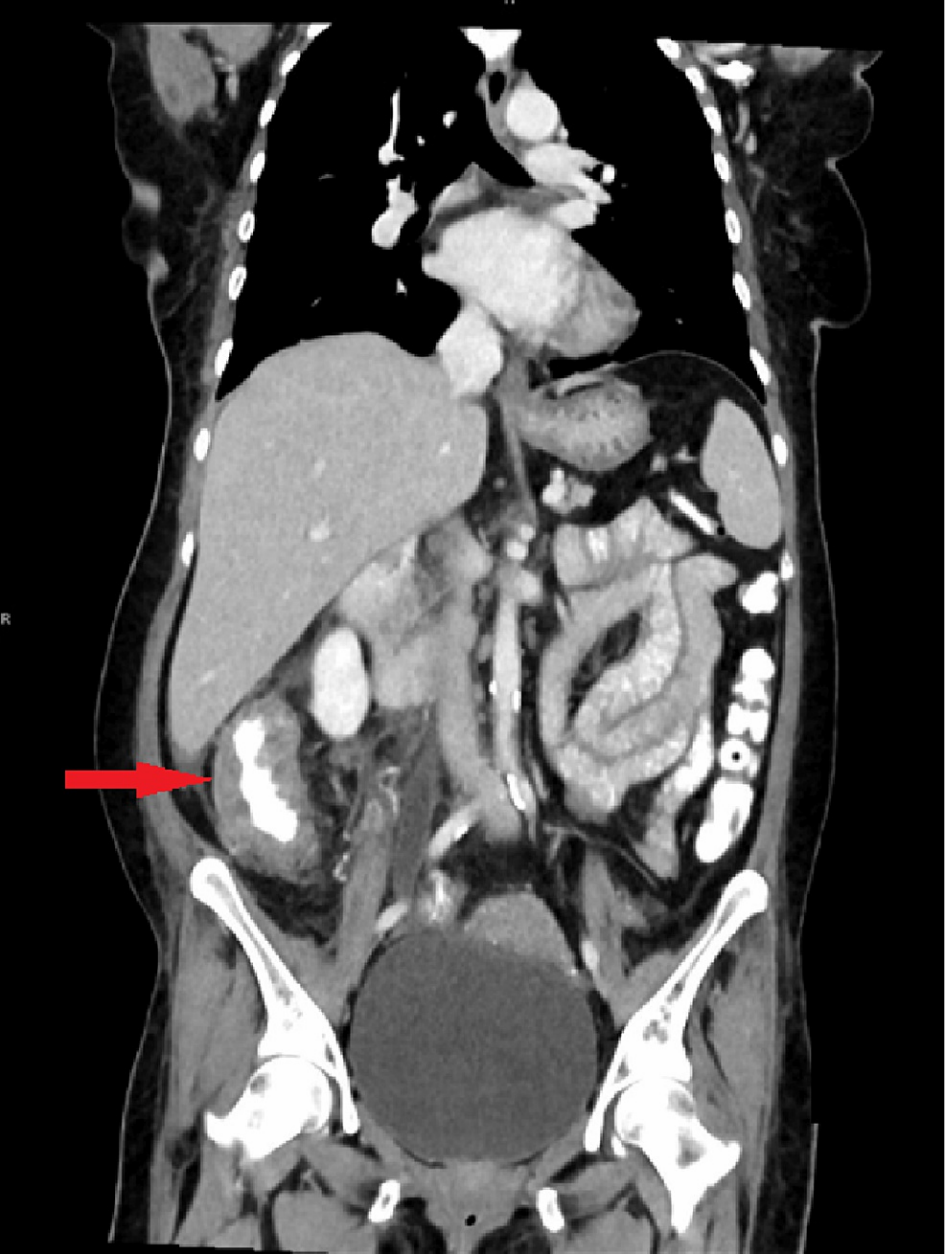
CT of the abdomen and pelvis showing perforated colon CT: computed tomography
